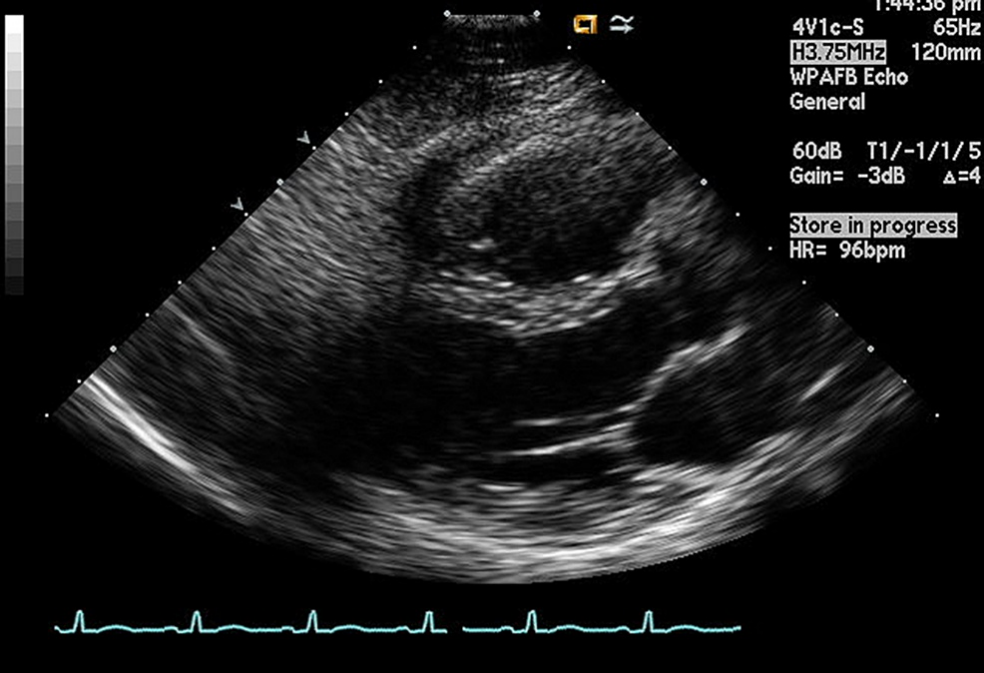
Right ventricle after recovery: follow-up echocardiogram done six months later showed normal right ventricle function
